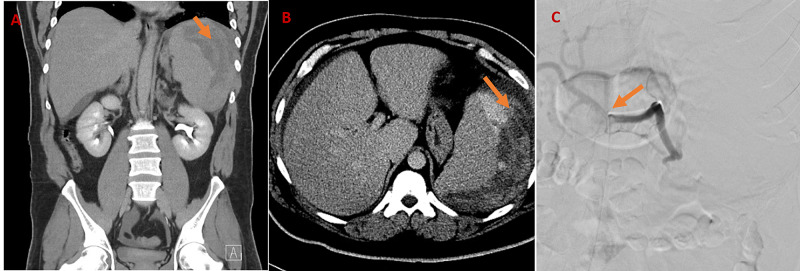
A, B: Computed tomography (CT) scan of abdomen and pelvis showing splenic hematoma; C: splenic angiogram with embolization
